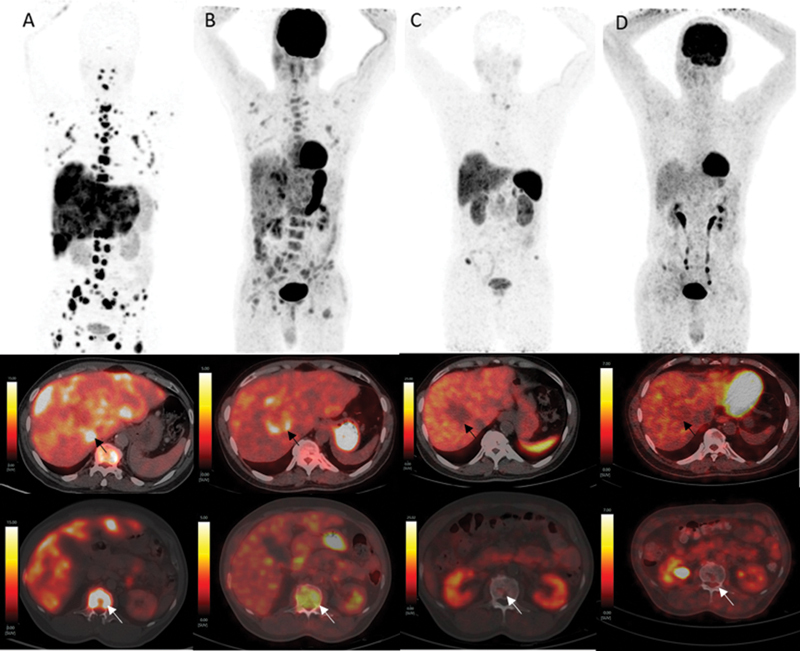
Dual-tracer positron emission tomography/computed tomography (PET/CT) ([68Ga]Ga-DOTATATE and [18F]fluorodeoxyglucose [FDG]) scans with maximum intensity projection (MIP) images showing both somatostatin receptor (SSTR) and [18F]FDG uptake in extensive liver and skeletal lesions at baseline (
